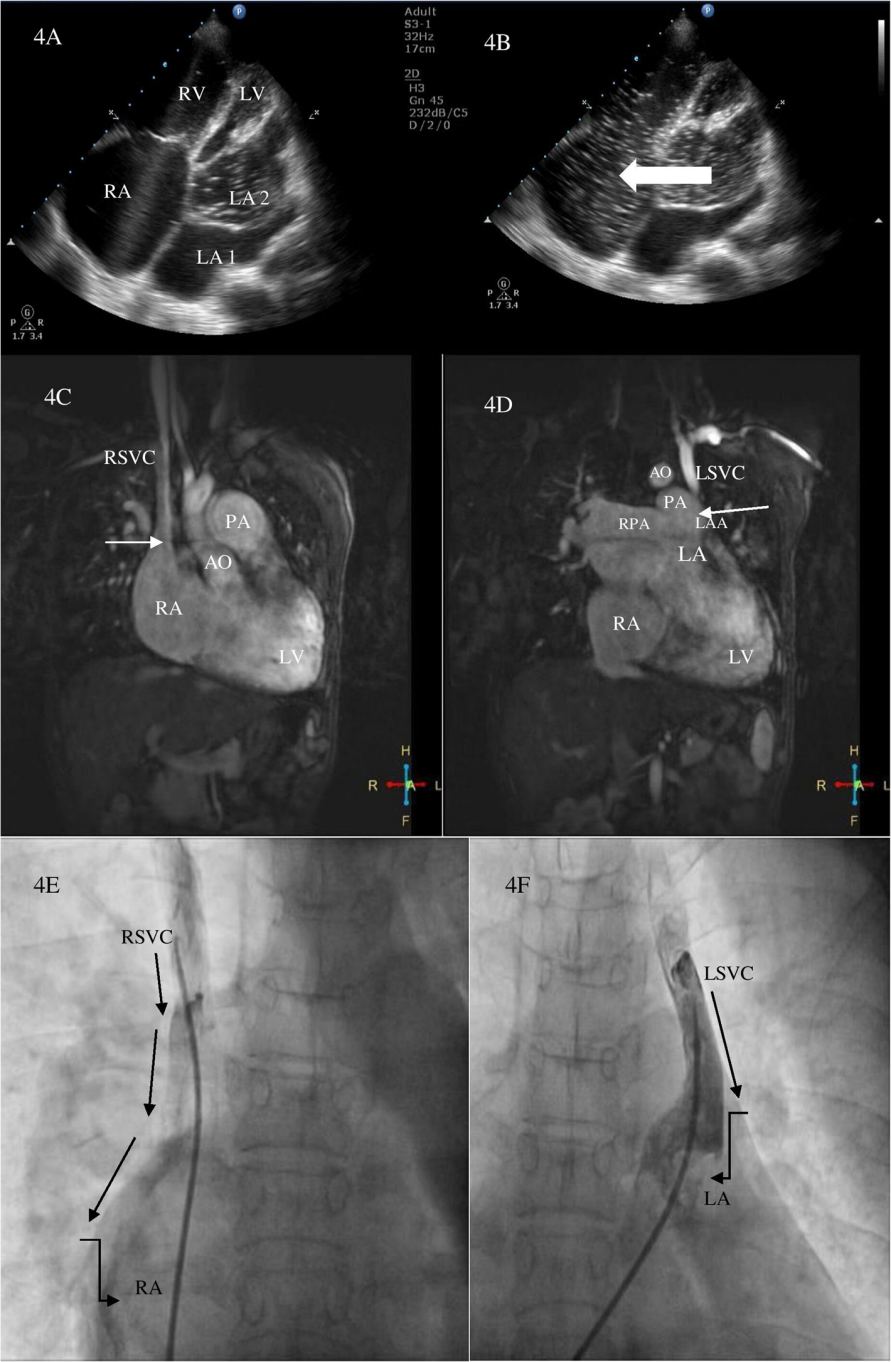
Contrast transthoracic echocardiography in apical 4 chamber view with contrast injected in left antecubital vein showing contrast 1st appearing in LA2 (A) and then progressing to right atrium through the ASD (arrow) (B). Cardiac MRI showing right superior vena cava draining into right atrium (arrow) (C) and left superior vena cava draining into left atrium (arrow) with absence of innominate vein (D). Fluoroscopic image with Berman catheter in right superior vena cava showing right superior vena cava draining into right atrium (arrows) (E) and left superior vena cava draining into left atrium (arrows) (F) confirming completely unroofed coronary sinus. * Indicates completely unroofed coronary sinus. LA, left atrium; RV, right ventricle; LV, left ventricle; RSVC, right superior vena cava; LSVC, left superior vena cava; LAA, left atrial appendage; AO, aorta; PA, pulmonary artery; RPA, right pulmonary artery
